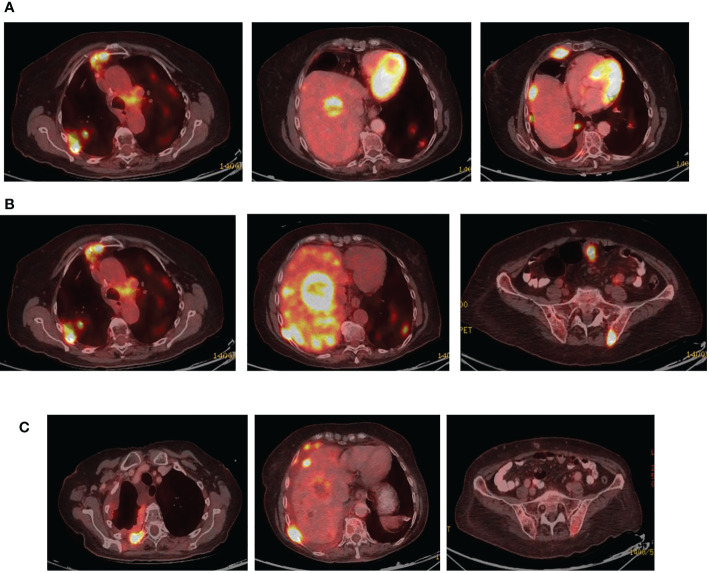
(A) Coronal PET-CT scan, from June 2021, showing new multiple lung and liver lesions with increased uptake of FDG-18, indicating further disease progression on osimertinib. (B) Two months later, August 2021, they demonstrated massive disease progression with lung, liver, and bone metastases following chemotherapy plus Osimertinib. (C) Three months later, November 2021, showed significantly decreased uptake of FDG-18, indicating partial response following adding dabrafenib plus trametinib to Osimertinib.
